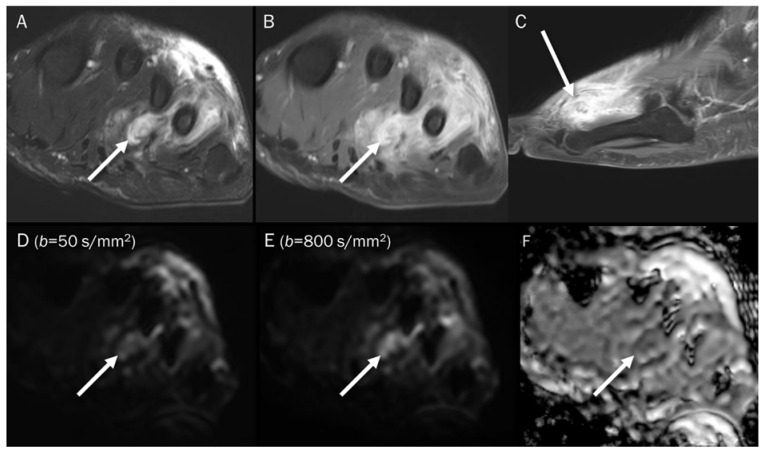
A 39-year-old male with foot dorsum pain. (A) Axial T2-weighted fat-suppressed image shows soft tissue swelling at the dorsal surface of the foot and interosseous muscle (arrow). (B,C) Axial and sagittal T1-weighted enhanced images show heterogeneous enhancement at the affected areas (arrows), suggesting phlegmon or abscess. (D,E) DWI with *b*-values of 50 and 800 s/mm^2^ exhibit progressive signal increase in the interosseous lesion (arrows) with (F) a reduced signal in the ADC map, suggesting “restricted” diffusivity, interpreted as an abscess.
